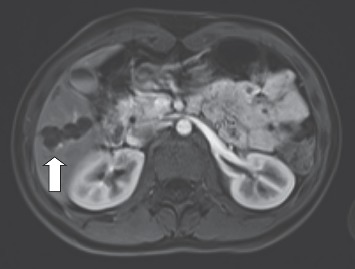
MRI scan of the patient no.2 after 1 month of treatment: 4 polycyclic cysts in S5/6, S7, and S8 of the liver.
